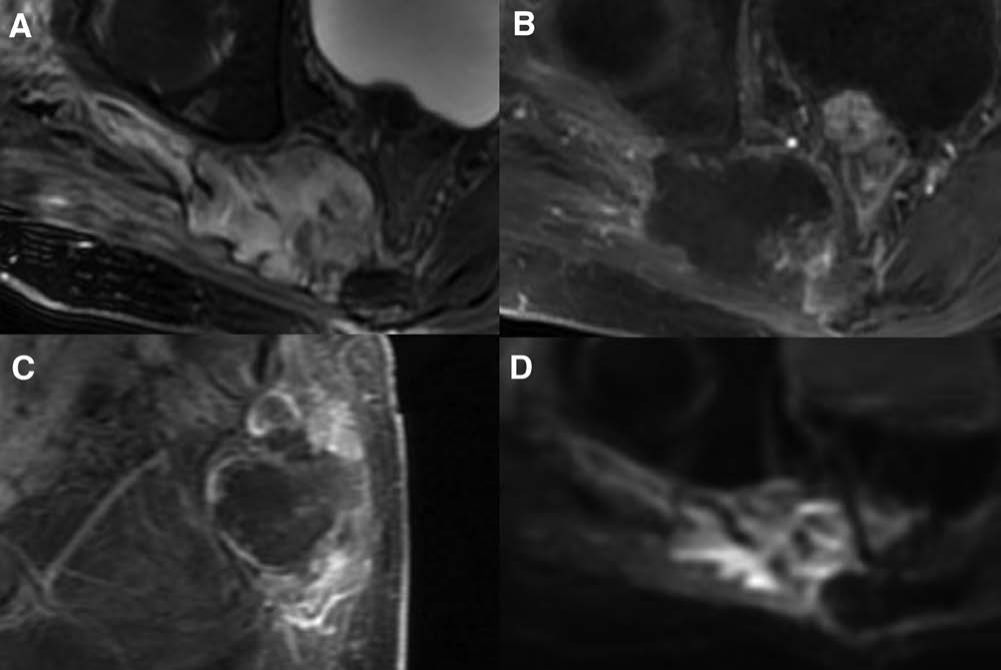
Postoperative MRI revealed infection at the surgical incision site. (A) MRI exhibited infection in the surgical area, which demonstrated mixed intensity on T2-weighted images with high intensity dominated. (B) Contrast-enhanced MR manifested significant enhancement of the lesion margin. (C) Sagittal view of contrast-enhanced MR manifested significant enhancement of the lesion margin. (D) The lesion demonstrated restricted diffusion on diffusion weighted images. MRI = magnetic resonance imaging.
